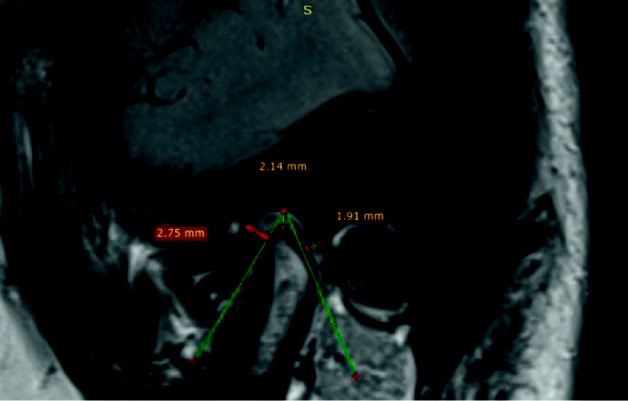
Measuring the TMJ space in MRI sagittal view (anterior joint space = 2.75 mm, central joint space = 2.14 mm, and posterior joint space = 1.91 mm).
